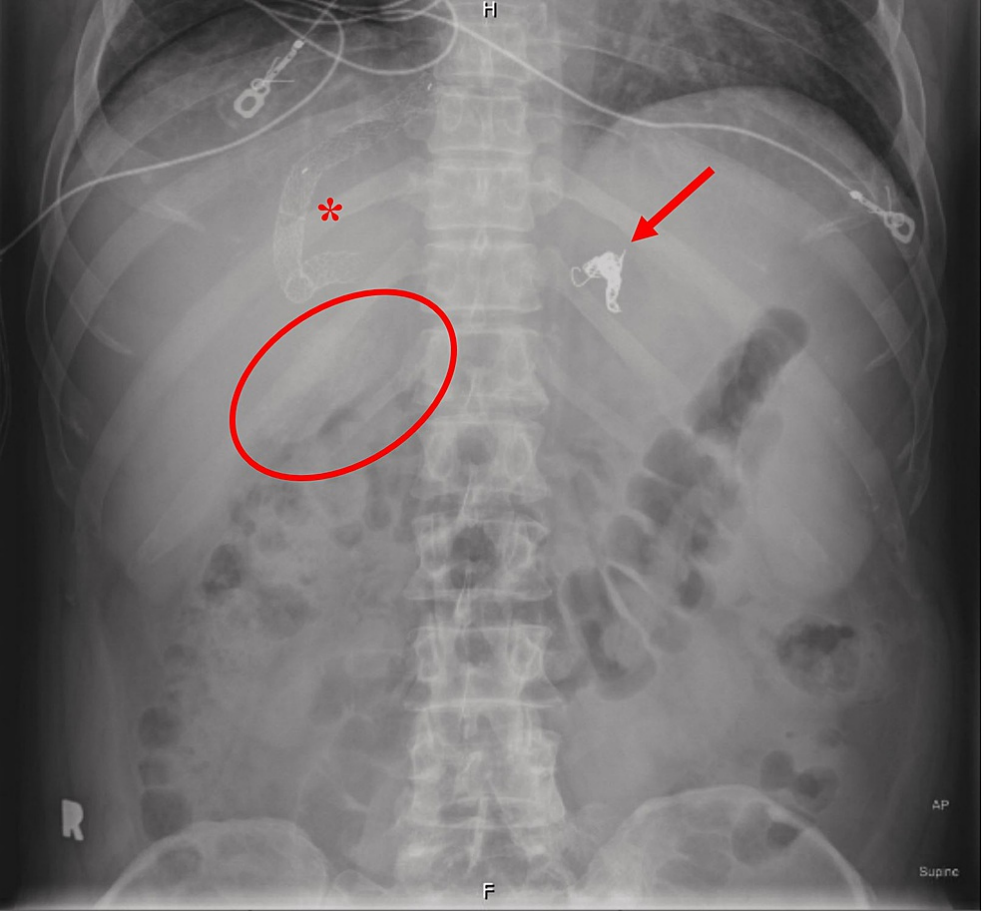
Abdominal radiograph showing diffusely radiopaque gallbladder (circle), a stent (asterisk) in the right upper quadrant, and embolization coils (arrow) in the left abdomen.
